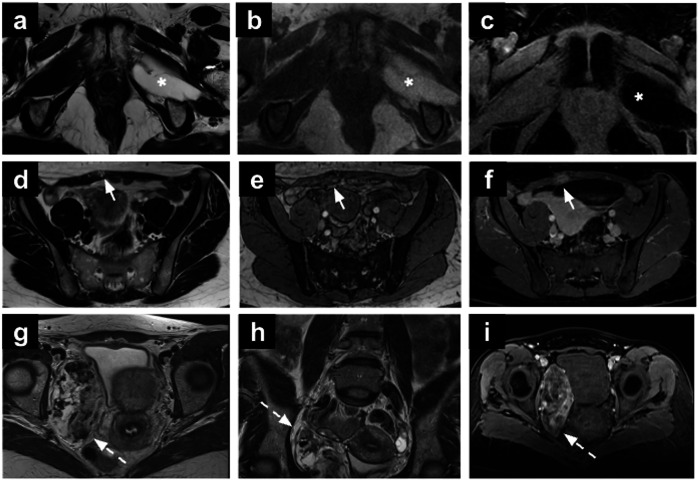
Lipoma (a–c). Vaginal cancer patient showing at the level of the left obturator externus, a large regular and homogeneous formation (white asterisks), appearing hyperintense on both T2-WI (a) and T1-WI (b) images and hypointense on fat-sat T1-WI (c), suggestive of intramuscular lipoma. Desmoid tumour (d–f). Axial T2-WI (d) and opposed-phase T1-WI (e) images from a 42-year-old woman undergoing MRI for adnexal mass characterisation showing a subtle heterogeneous signal mass within the right rectus abdominis muscle, better depicted as hypervascular on post-contrast axial T1-WI (f), pathologically confirmed as desmoid tumour. Aggressive angiomyxoma (g–i). Axial (g) and coronal (h) T2-WI show a hyperintense mass (dotted arrows) with swirled appearance and hypointense linear foci within the right hemipelvis, heterogeneously enhancing on post-contrast axial T1-WI (i), in keeping with aggressive angiomyxoma in a 57-year-old woman. WI, weighted images
